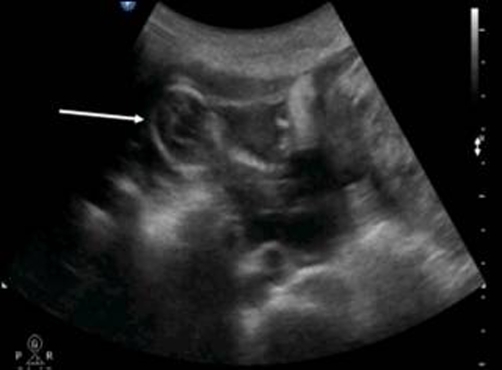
Pelvic ultrasound scanning. Diffuse free intraperitoneal fluid was seen around the fetus and small bowel loops.
